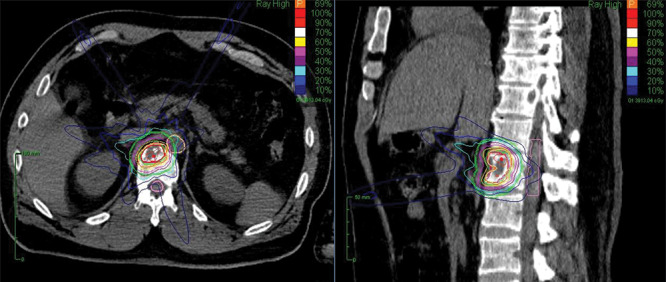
The left and right panels are axial and sagittal CT images, respectively, with contouring for planning SRT.
